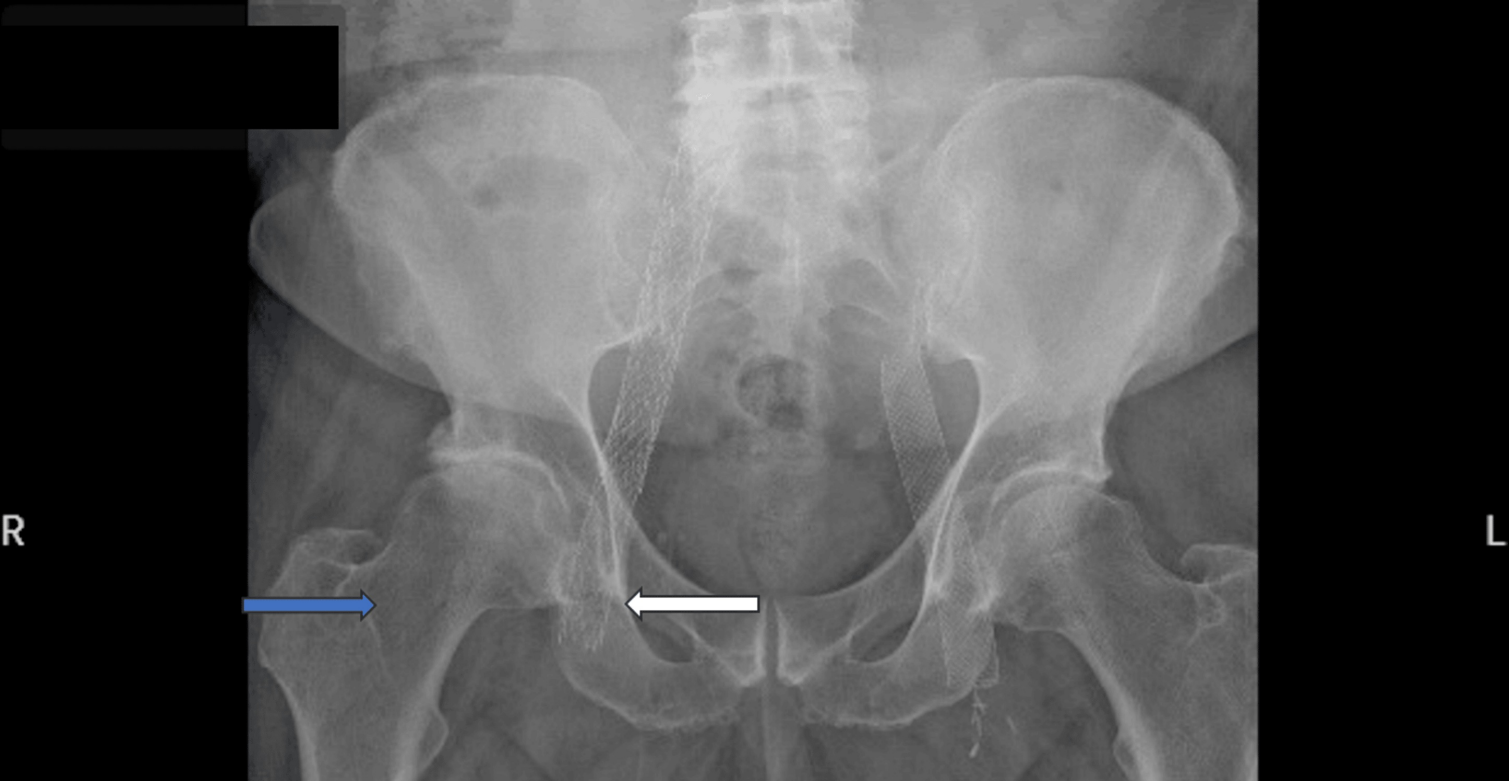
X-ray showing the proximity of the iliac venous stent (white arrow) to the surgical site (blue arrow).
